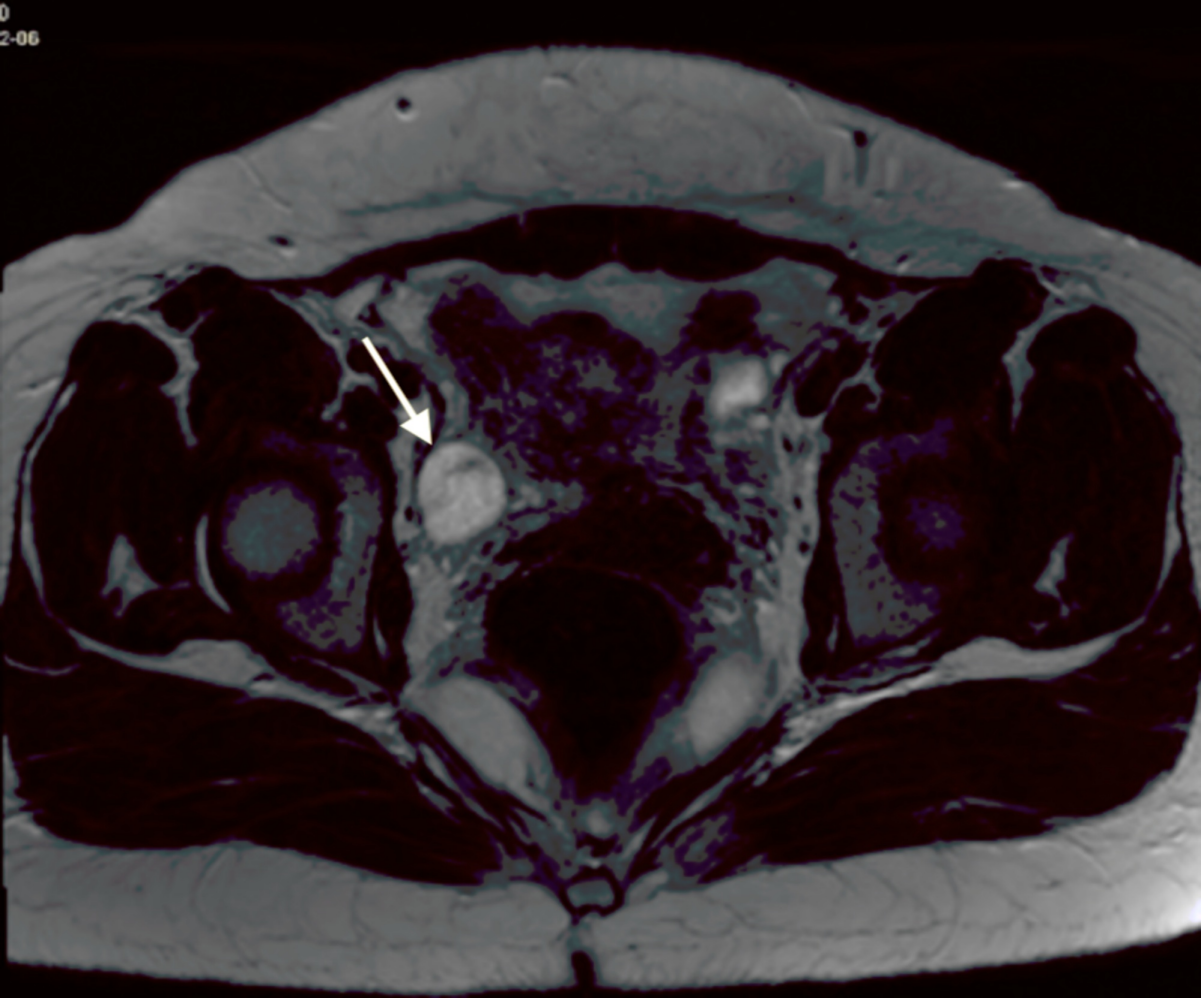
Axial MRI Pelvis with IV Contrast
